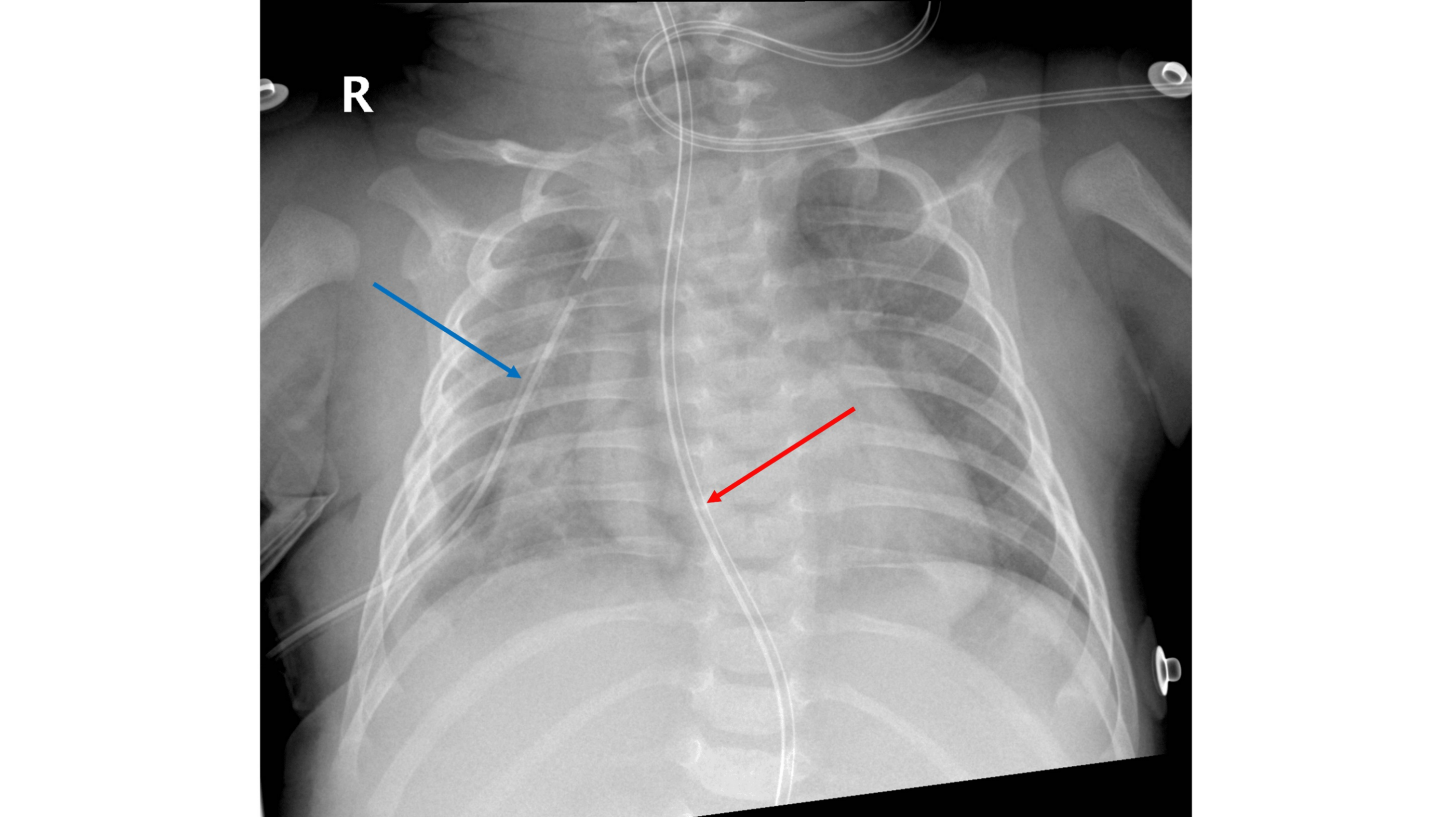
Postoperative chest X-ray showing the successful passage of the nasogastric tube along with a correct placement of the chest drain. The red arrow points to the nasogastric tube, and the blue arrow points to the chest drain.
